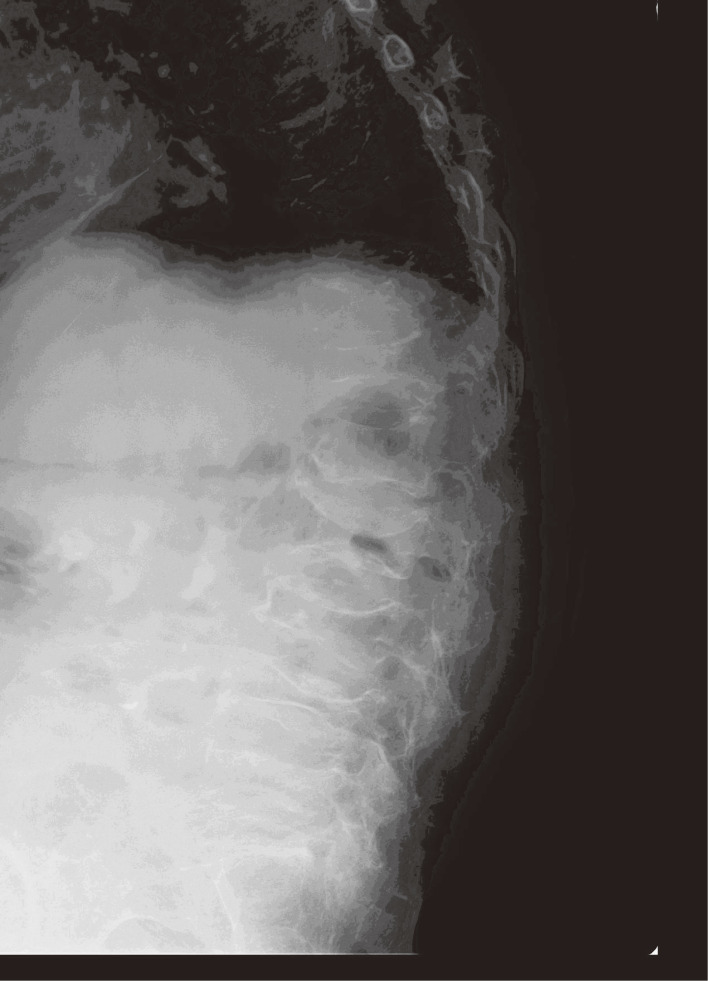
An X-ray photograph of thoracic and lumbar vertebrae from the side.
